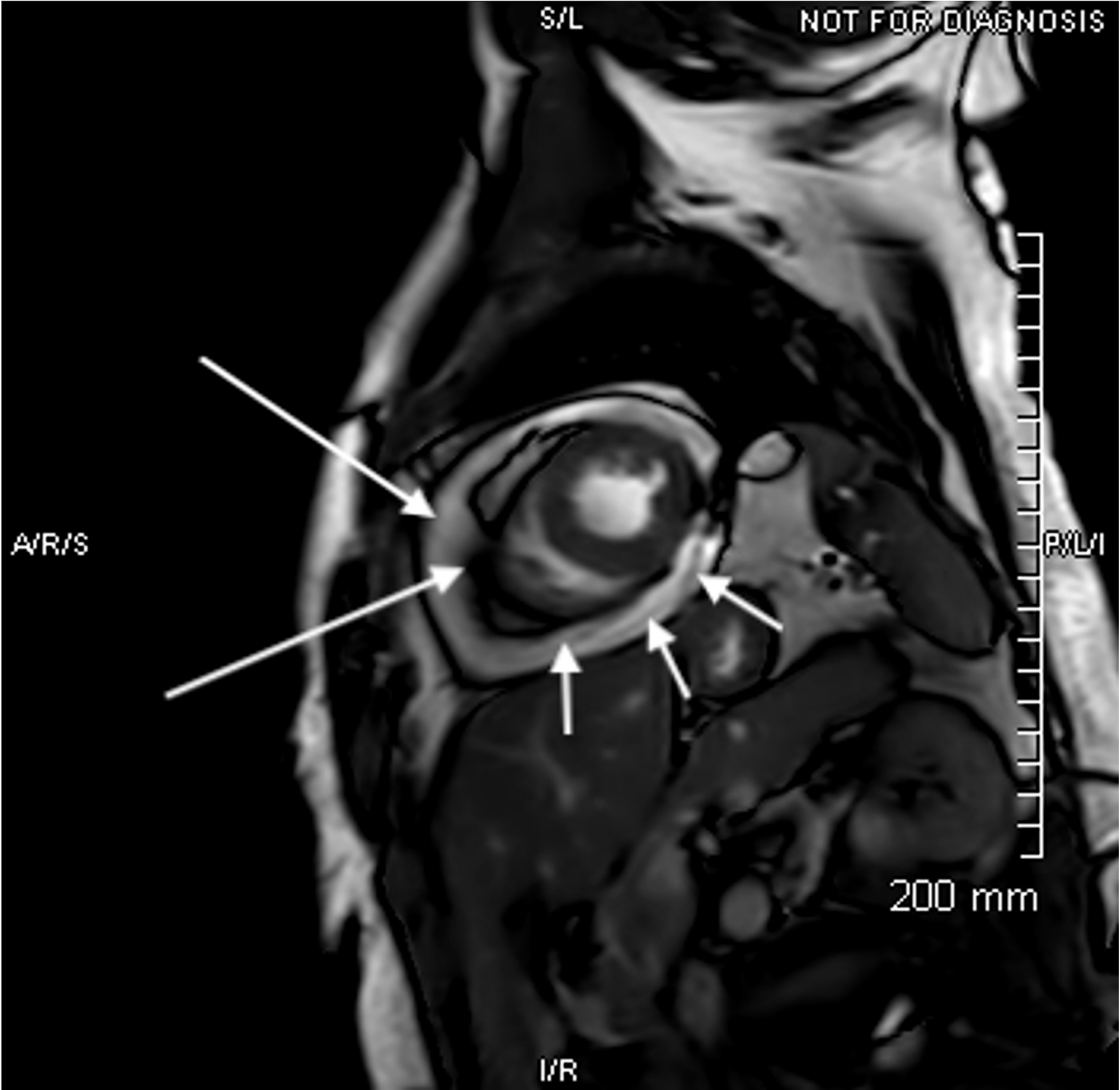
Cardiac MRI. Short axis steady-state free precession image demonstrating large pericardial effusion (short arrows) with blooming along the visceral and parietal pericardium (long arrows) due to hemosiderin deposition in the setting of a hemorrhagic effusion.
